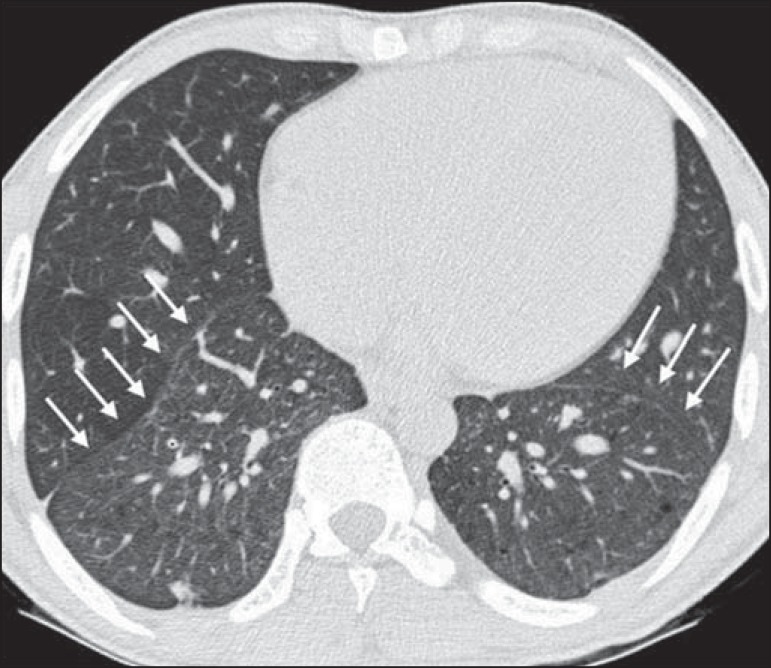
Axial chest CT scan showing significant volume reduction in the lower
